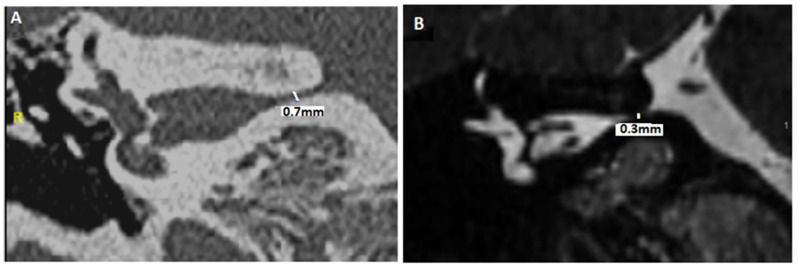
Assessment of the diameter of the internal auditory canal in the coronal plane. (A): HRCT of the temporal bone. (B): MRI, sequence: T2 (high resolution). R: right side.
